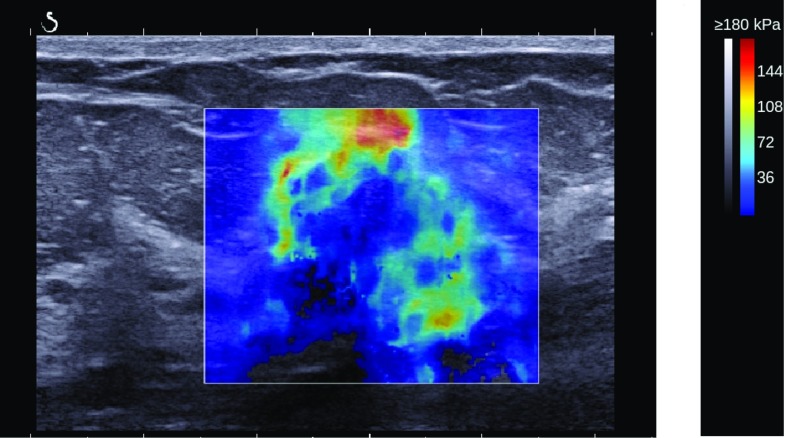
SWE image of a malignant solid breast lesion. The elasticity values are represented as a colour map overlaying the greyscale B-mode ultrasound image
